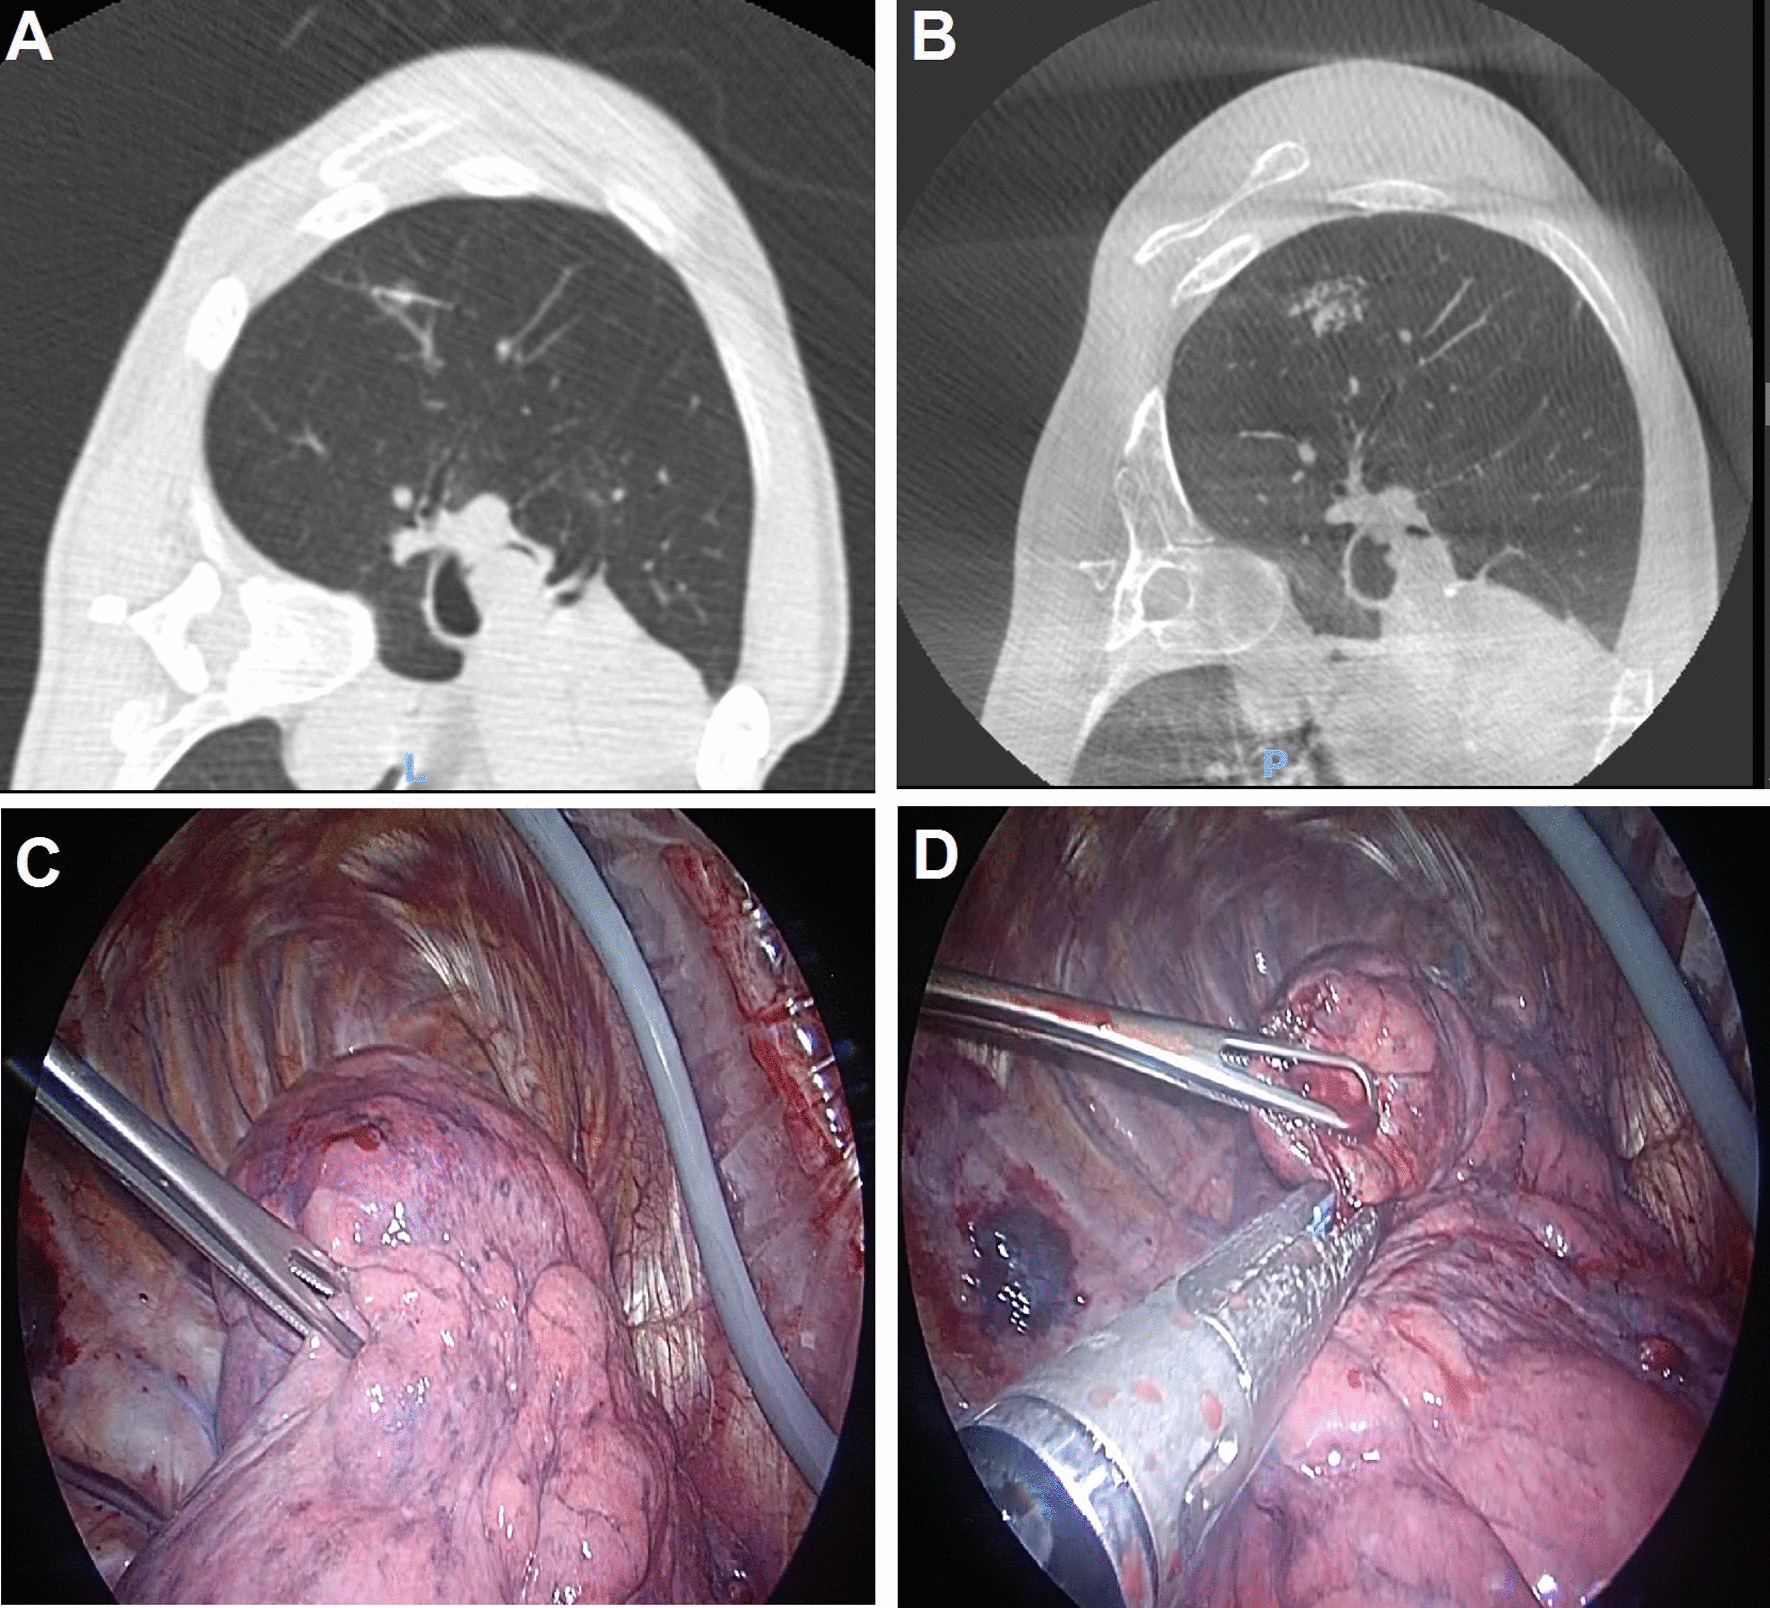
Intra-procedural and intra-operative images depicting nodule localisation and subsequent resection A CT scan showing that the hookwire placed in IR suite was within lesion of interest prior to transfer to hybrid OR. B Pre-operative cone-beam CT showing complete hookwire dislodgement, but the resulting surface hemorrhage could be used to guide subsequent resection of the lesion of interest. C Intra-operative view of the surface hemorrhage seen on pre-operative cone beam CT. D Wedge resection of the lesion of interest performed using the previously seen surface hemorrhage as a landmark, and depth of resection was guided by measuring depth of lesion of interest from the surface hemorrhage seen on pre-operative cone-beam CT
